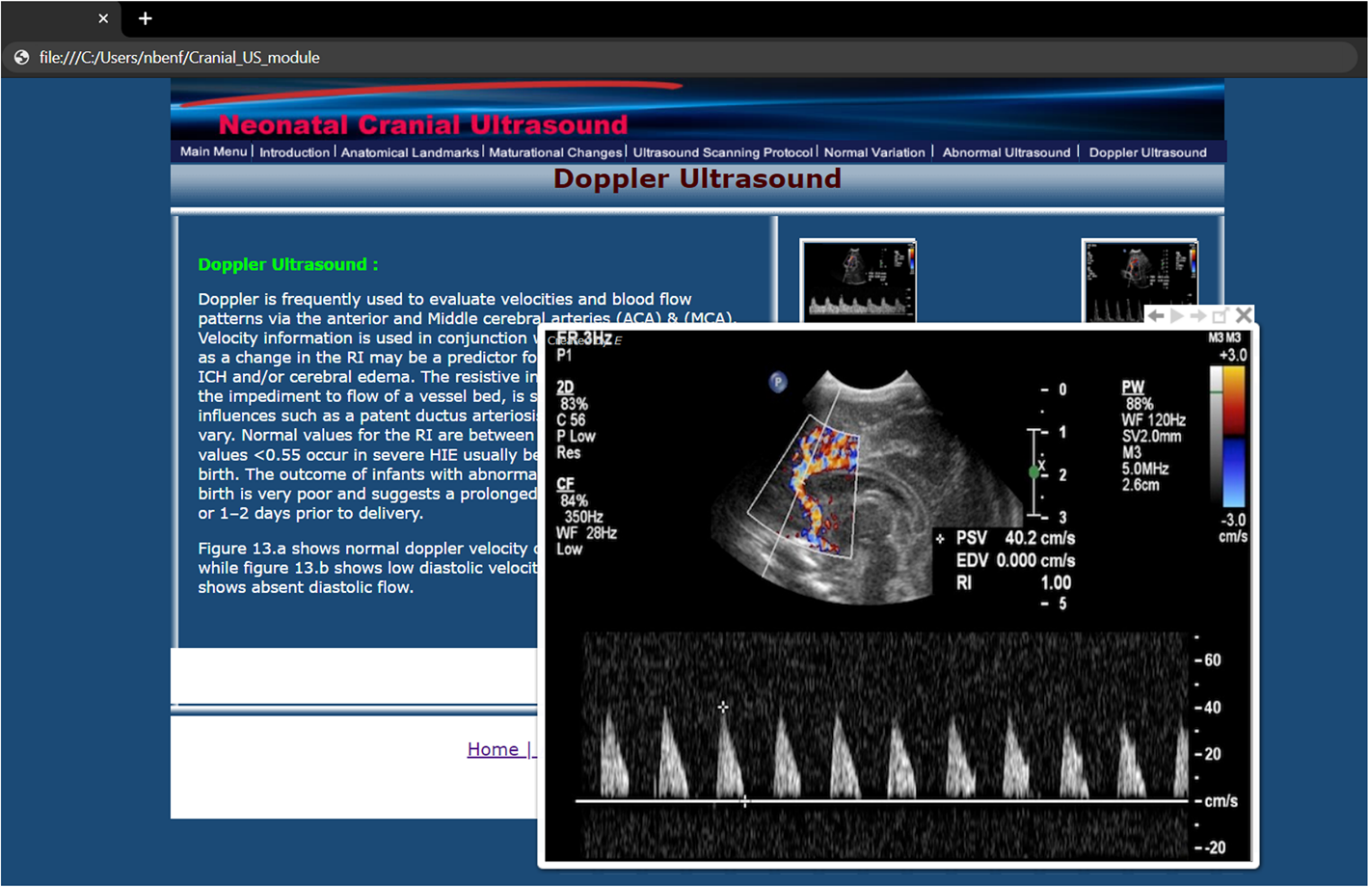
Screenshot of the Doppler CUS page Legend: The images are displayed as a thumbnail and will enlarge once the learner clicks on it. Enlarging more than one image allows for comparison
